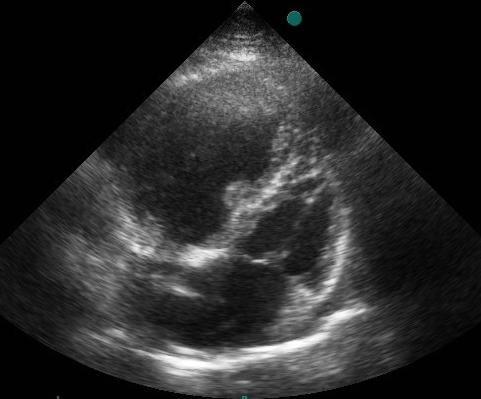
Parasternal transthoracic echocardiographic view demonstrating and confirming the ventricular pacing lead going from the right atrium to the right ventricle with its tip located in the apical position.
